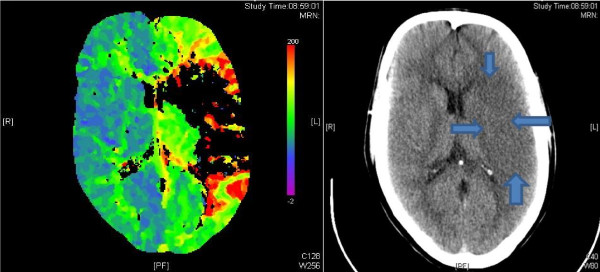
Showing a perfusion scan and a CT scan of a patient with a Left middle cerebral artery infarct [12][modified from reference [13]].
